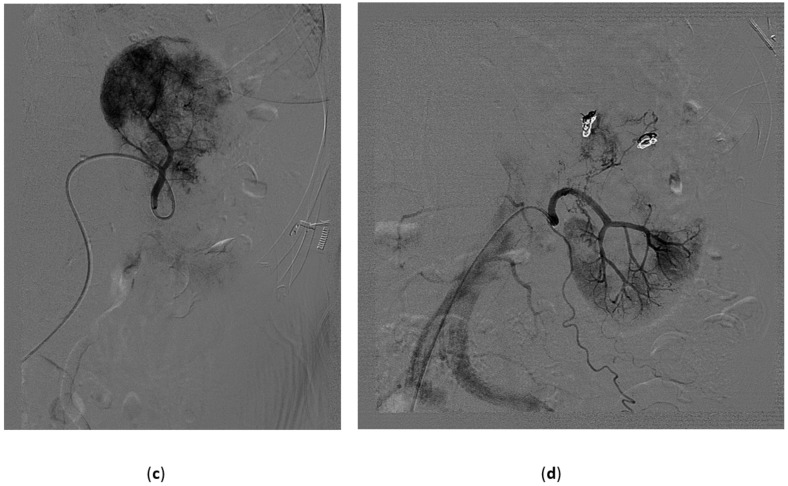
Malignant renal tumor. 91-year-old female with hematuria, anemia, and left flank pain. Palliative embolization of the left kidney was scheduled. (a,b) Contrast-enhanced CT (axial and sagittal plane) shows a huge not homogeneous mass of the superior half of the left kidney; (c) Selective angiography: rich vascularized renal lesion. (d) Angiogram (after embolization): the lesion is no longer visible; the inferior pole of the left kidney shows normal aspect.
